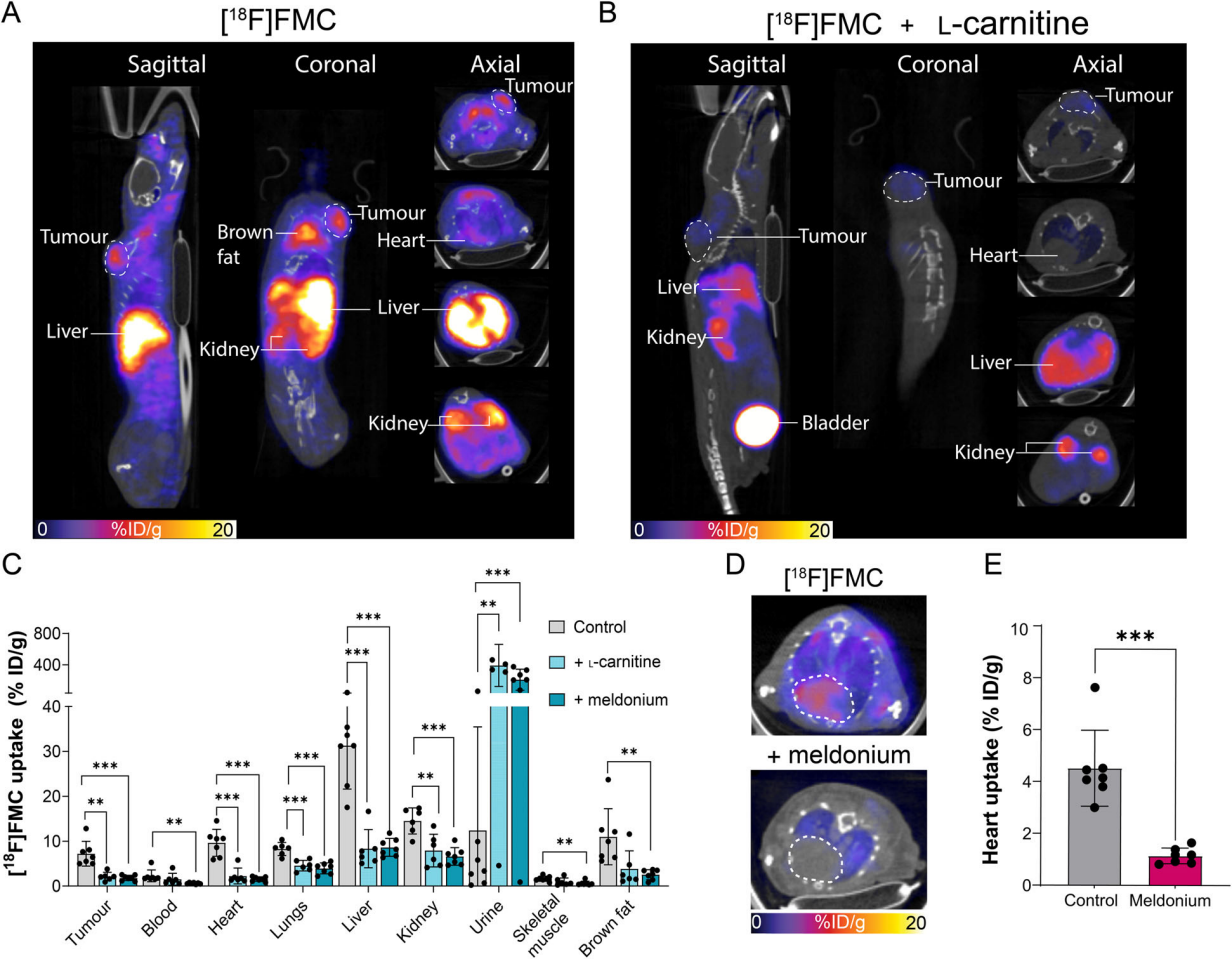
Imaging aberrant carnitine utilisation in NSCLC with [^18^F]FMC PET. A and B) Representative 120 min sagittal, coronal, and axial PET/CT images of [^18^F]FMC in a human NSCLC (H460) subcutaneous xenograft tumour model (A), with co‐injection of carnitine (50 mg kg^−1^; B). C) Ex vivo biodistribution of [^18^F]FMC in selected tissues at 2 h post‐injection in the presence and absence of carnitine and meldonium (*n* = 6‐7). Analysis was performed by one‐way analysis of variance (ANOVA) followed by *t*‐tests, multiple comparison correction (Dunnett's method). D) Representative axial PET/CT images of heart [^18^F]FMC uptake at 120 min with and without co‐injection of meldonium. An unpaired two‐tailed Student's *t*‐test with Welch's correction was performed (*n* = 7). E) Image‐derived quantification of [^18^F]FMC heart uptake at 120 min in the presence and absence of meldonium. Data are presented as mean ± SD. ^*^
